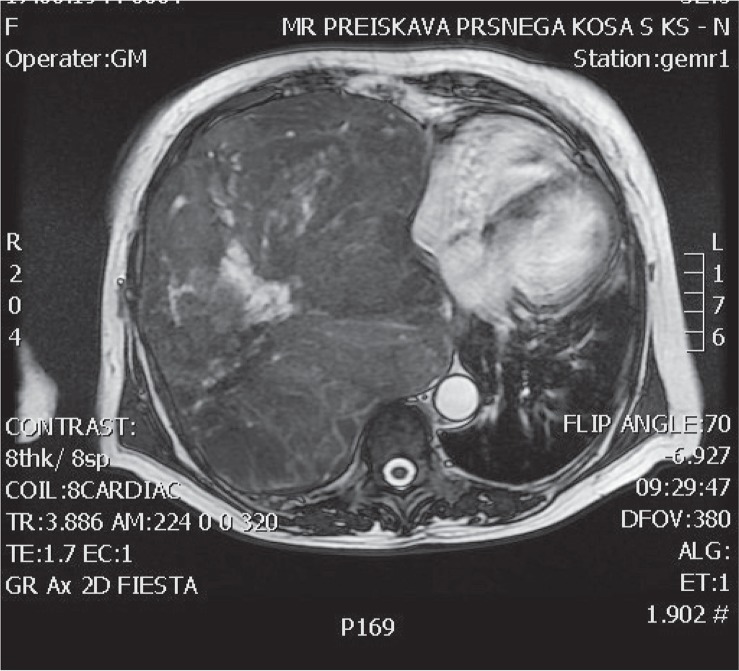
Same tumour displayed using MRI - no signs of overgrowth in the structure of the mediastinum.
